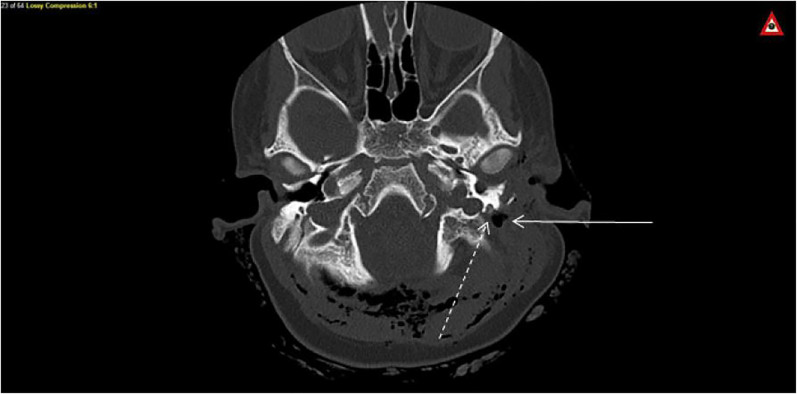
Computed tomography demonstrating soft tissue swelling (solid arrow) abutting the hyperdense area of the facial nerve, sounded by bone (dashed arrow)
